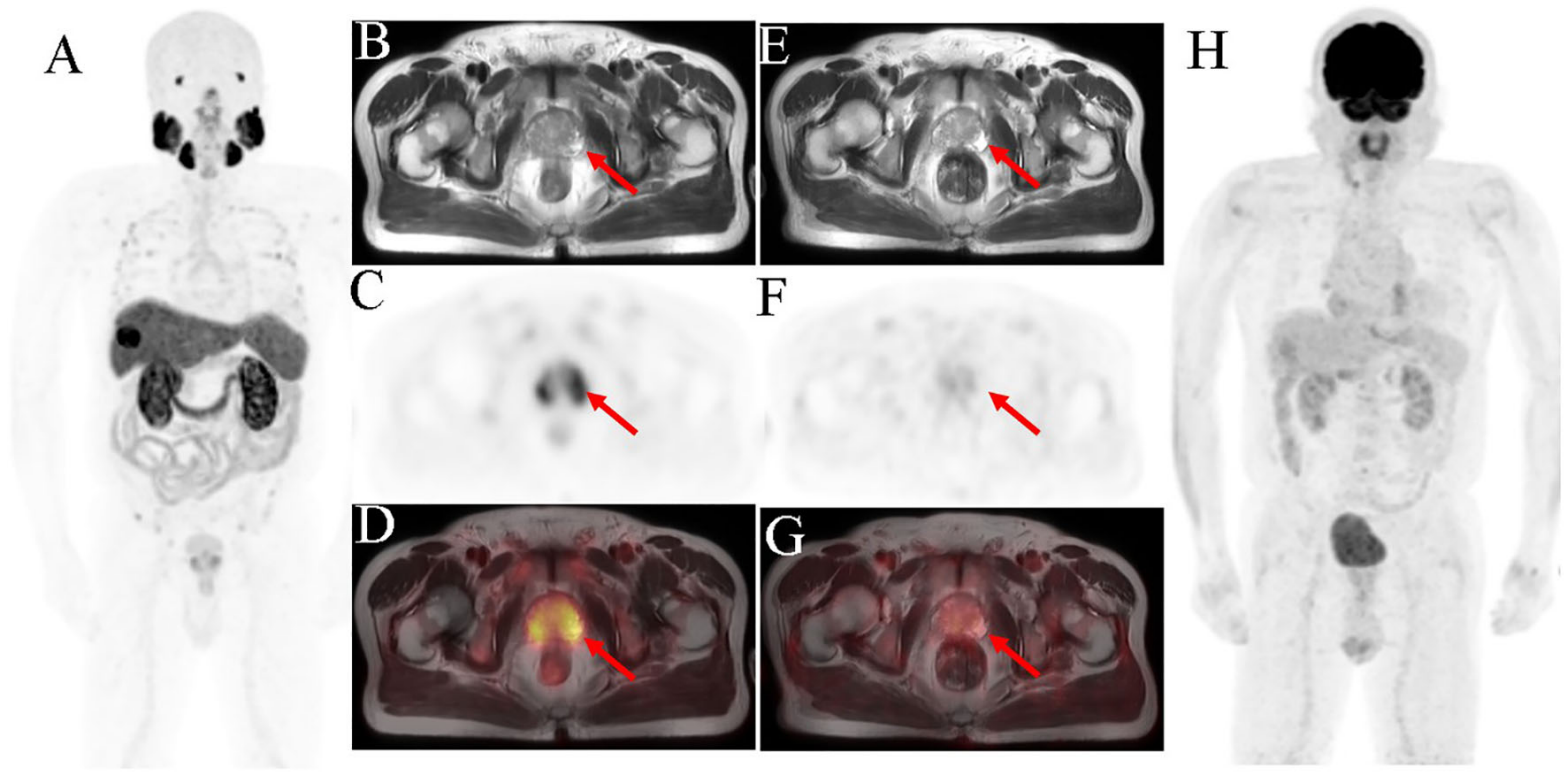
Prostate biopsy conducted in a 71-year-old man owing to high PSA level (5.54 ng/ml) and difficult, frequent, and urgent urination. The pathological result showed acinar adenocarcinoma, and the Gleason score was 6. ^18^F-PSMA-1007 PET/MRI imaging was performed for initial staging. The scan [(A) MIP image; (B) axial T2WI-MRI; (C) axial PET and (D) fused axial PET/MRI] shows high PSMA uptake in the bilateral peripheral zone of the prostate (SUVmax was 5.53). The ^18^F-FDG PET/MRI scan [(E) axial T2WI-MRI; (F) axial PET; (G) fused axial PET/MRI and (H) MIP image] shows no abnormal radiotracer concentration in prostate. The red arrow indicates the prostate.
